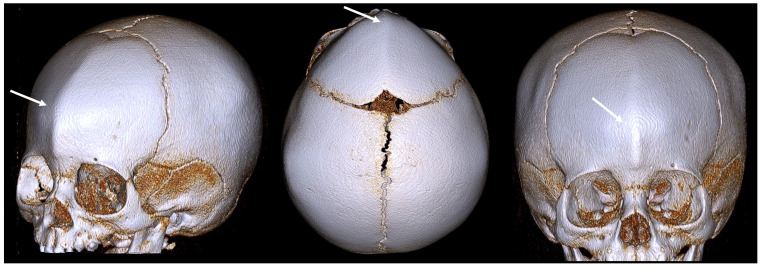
A 3D CT example of metopic CRS (trigonocephaly) with a triangular keel-shaped forehead, hypotelorism, and a midline ridge along the forehead. The white arrow indicates an abnormally fused suture.
